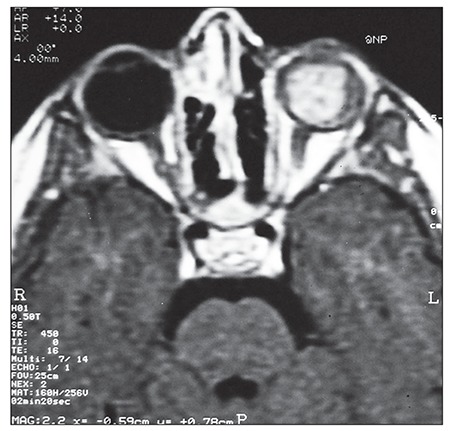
Orbital MRI shows a 2 x 1.5-cm mass lesion with irregular borders completely filling the bulbus oculi.
